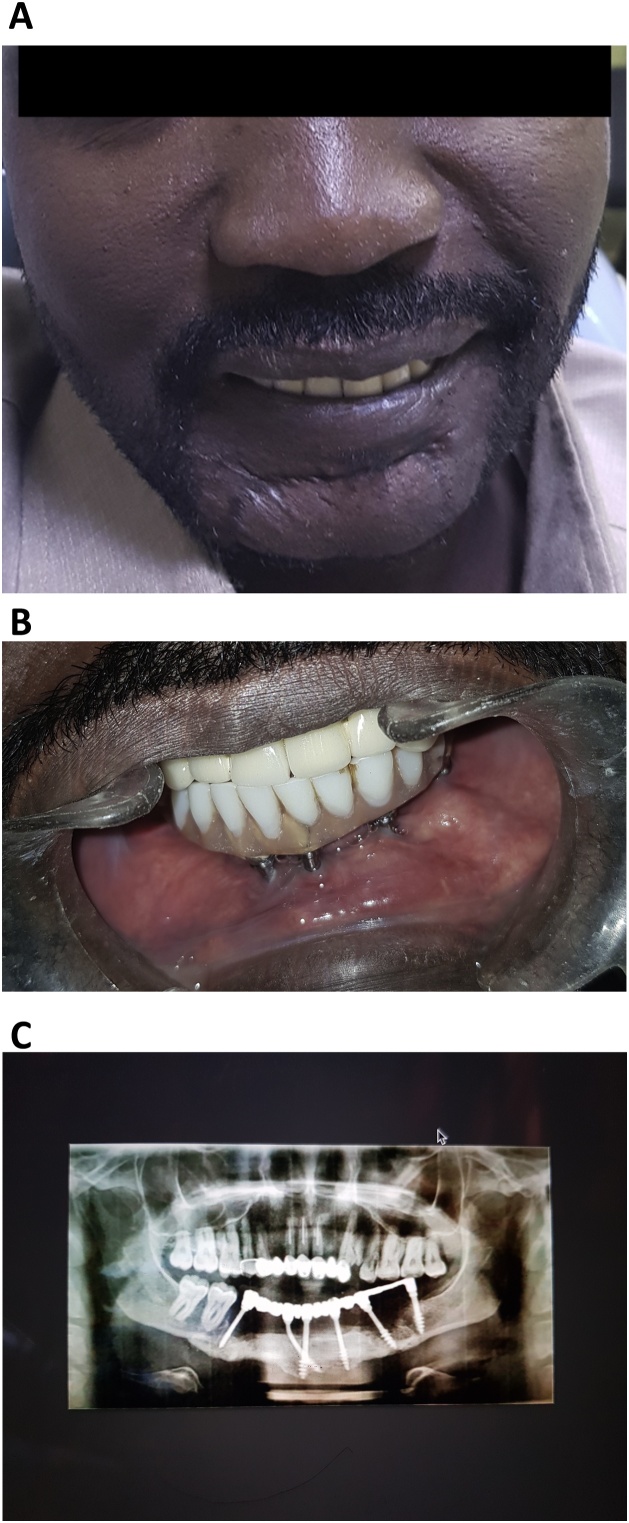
Follow-up images of the immediately loaded basal implant-supported fixed prostheses fabricated for a 32-year-old man with a gunshot defect. A. The frontal view of the patient after 5 years of follow-up. B. The intraoral view of the patient depicting his clinical presentation exhibiting optimal peri-implant oral health. C. A panoramic radiograph showing the maxillary and mandibular prostheses after 5 years of function.
